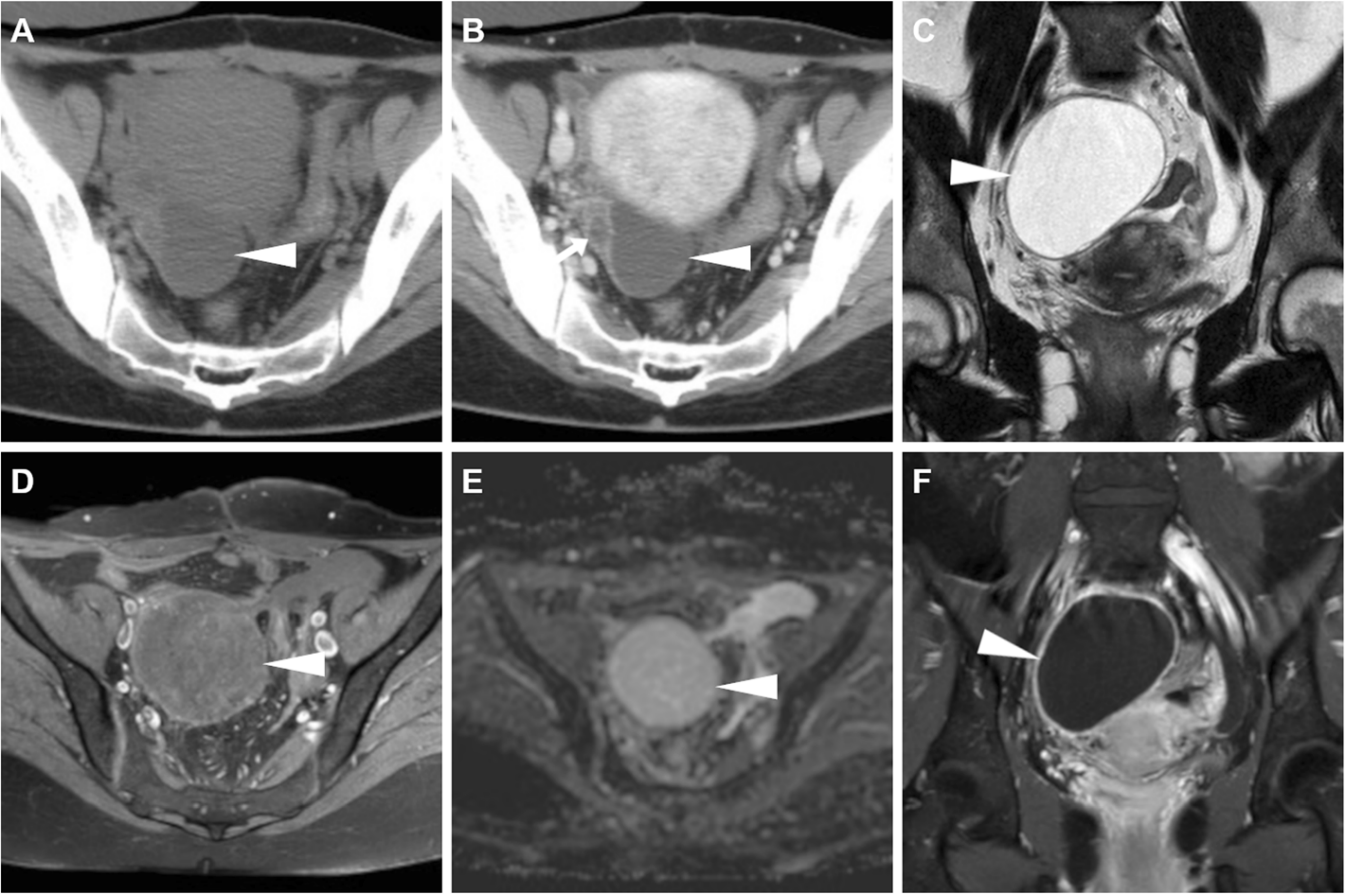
A surgically proven, progressively enlarging ovarian cyst. Initial unenhanced (a) and post-contrast (b) CT images depicted a 4 × 3 cm non-enhancing retrouterine cystic lesion (arrowheads) demarcated by a thin regular wall. Weeks later, MRI showed cyst enlargement with homogeneous T2-weighted fluid signal (c), without evidence of blood on precontrast fat-suppressed T1-weighted sequence (d), with unrestricted diffusion on apparent diffusion coefficient map (e) and thin uniform enhancing wall on post-gadolinium acquisition (f)
